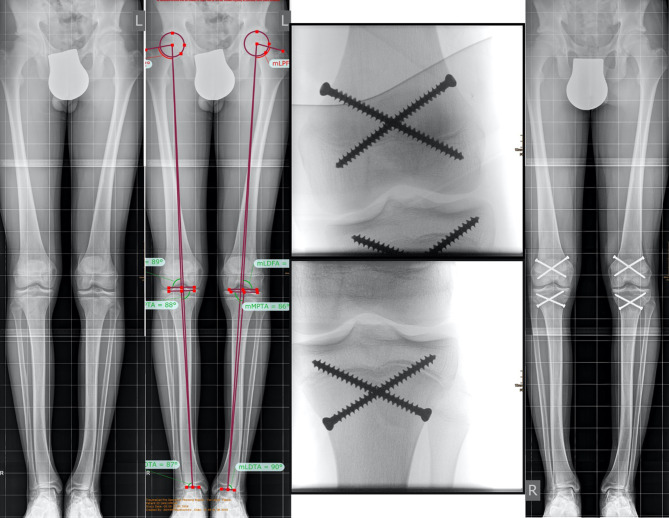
Example of bilateral percutaneous epiphysiodesis using transphyseal screws (PETS) to reduce excessive predicted height. a Full standing anterior/posterior bilateral lower extremity radiograph of a boy with familial tall stature. b Preoperative limb alignment analysis. Growth prediction resulted in a predicted final height of 208 cm. c, d Intraoperative a/p x-rays of the left distal femur and tibia; e follow-up X‑ray after completed growth with a final height of 197.5 cm
